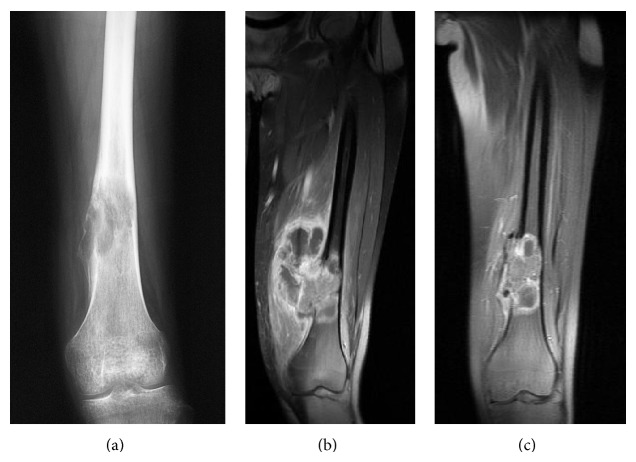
A plain radiograph of the left distal femur demonstrates an osteolytic lesion with destruction of the cortex and intratumoral ossification (a). Coronal MR images demonstrate a large extraosseous mass with destruction of the cortex (b). Reduction in size of the tumor was noted after preoperative chemotherapy (c).
